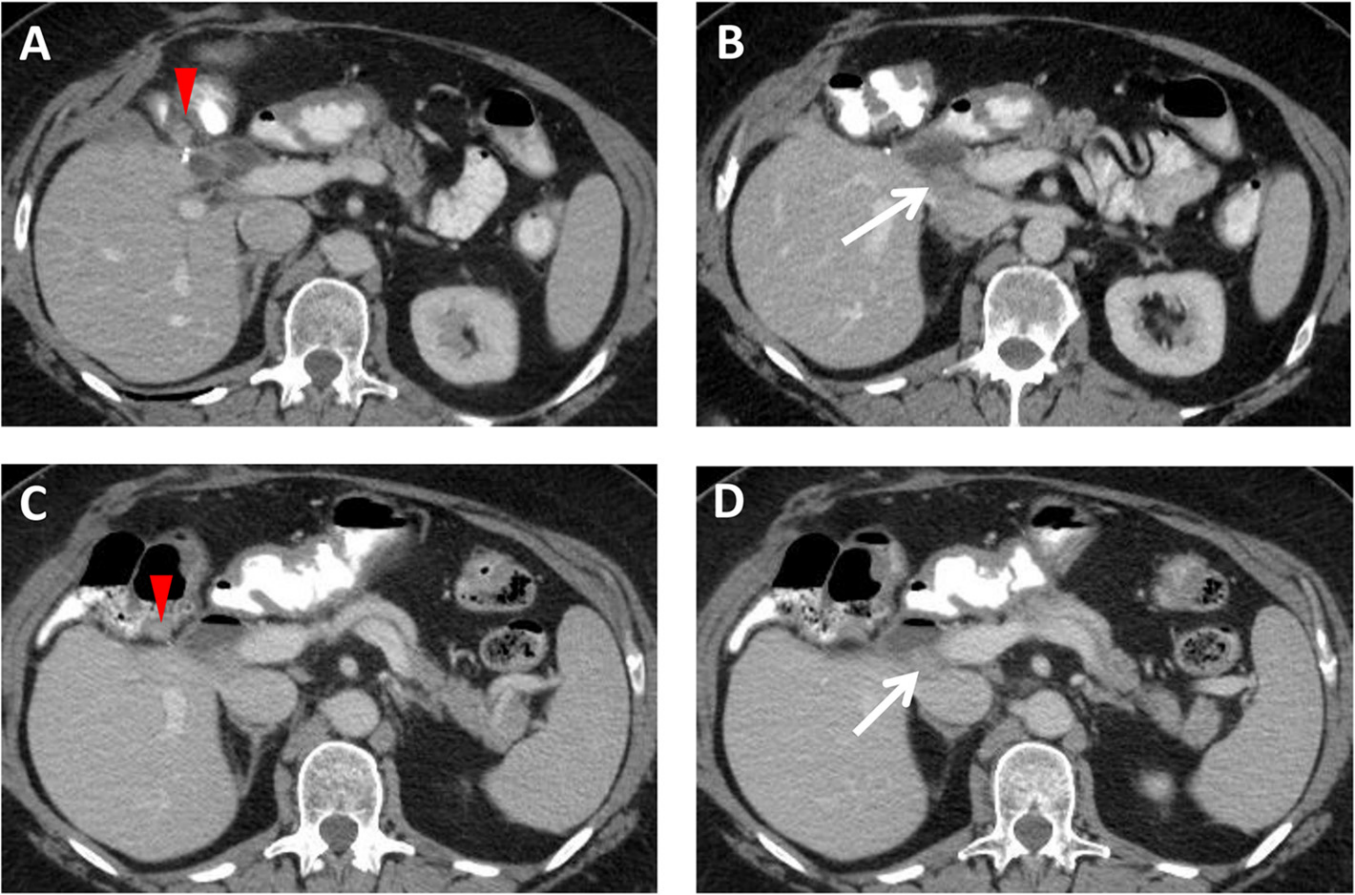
A 64-year-old female with recurrent gallbladder carcinoma. Axial contrast-enhanced CT images demonstrate a a 1.2-cm nodule (*arrowhead*) in the gallbladder fossa adjacent to the hepatic flexure and b a 1.7-cm nodule (*arrow*) in the portocaval region. Both nodules were new from the postoperative scan (following resection of recurrent tumor in the gallbladder fossa), in keeping with recurrence. c, d Thirteen months later, both nodules are stable after treatment with trastuzumab
